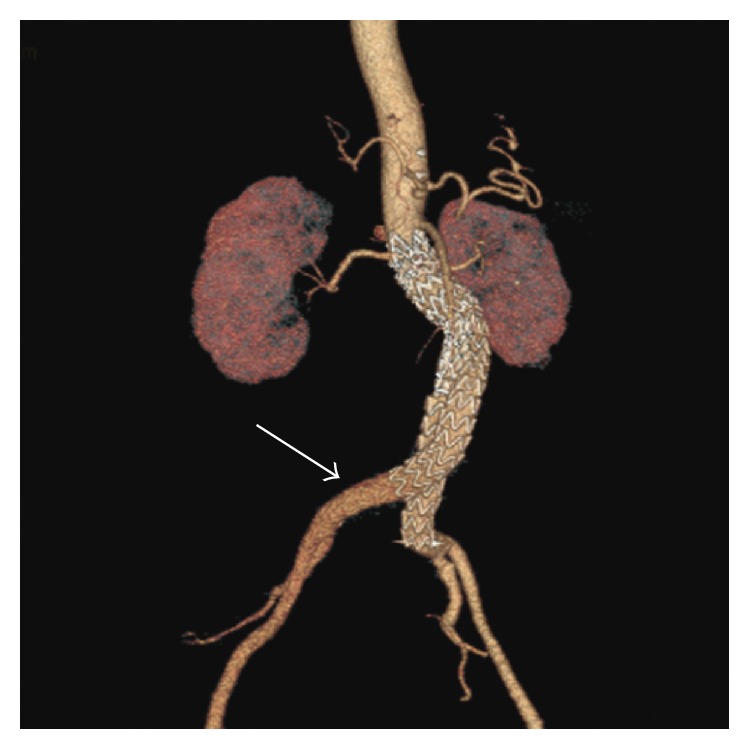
Computed tomography angiography performed on postoperative day 2 demonstrating the proximal migration of the right endograft limb (arrow).
